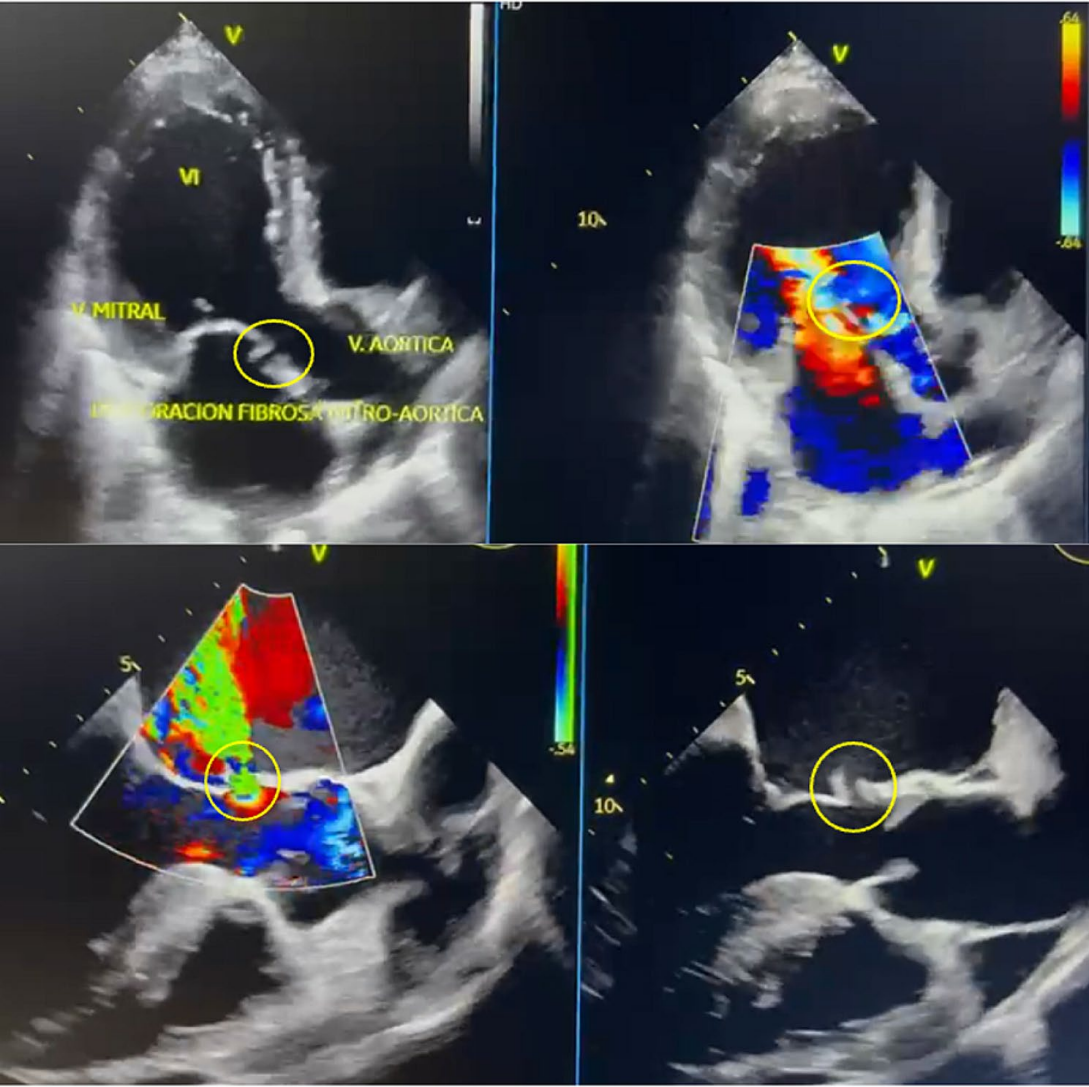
Preoperative transesophageal echocardiogram (A) Two-chamber apical view. Evidence of fistula in the mitro-aortic fibrous portion (yellow circle) with competitive flow. (B) Longitudinal or long-axis view. Evidence of fistula in the mitro-aortic fibrous portion (yellow circle) with competitive flow.
